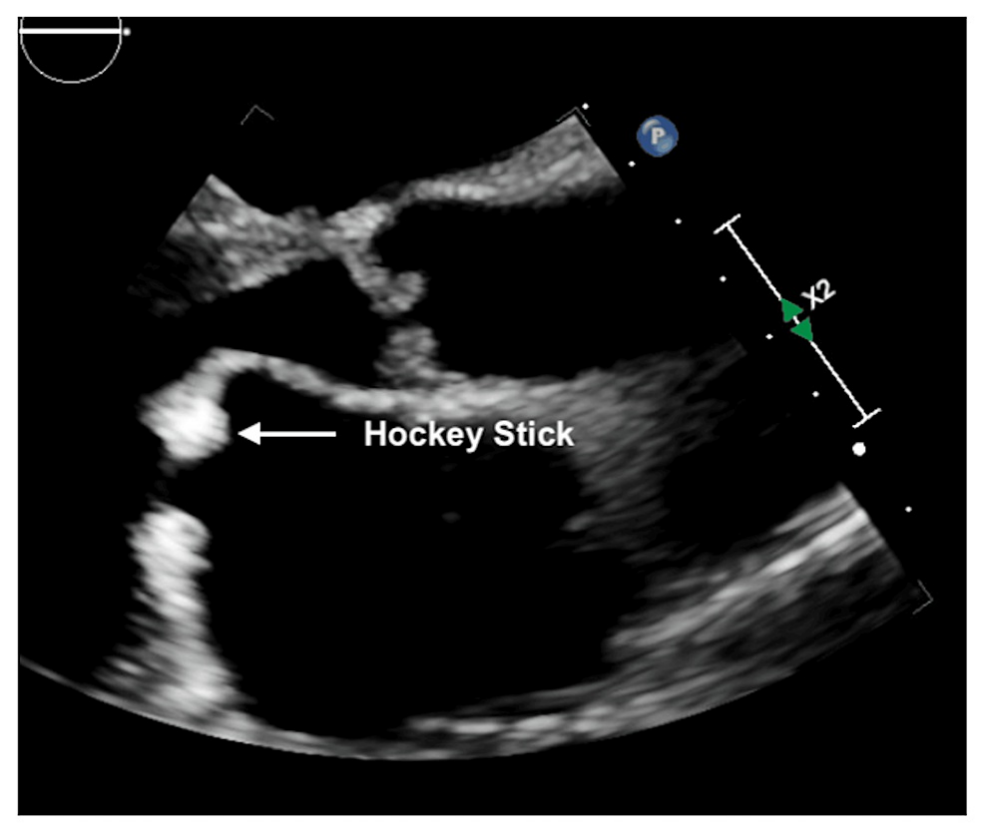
Transthoracic echocardiography parasternal long-axis view showing hockey stick of anterior and posterior mitral valve leaflets
